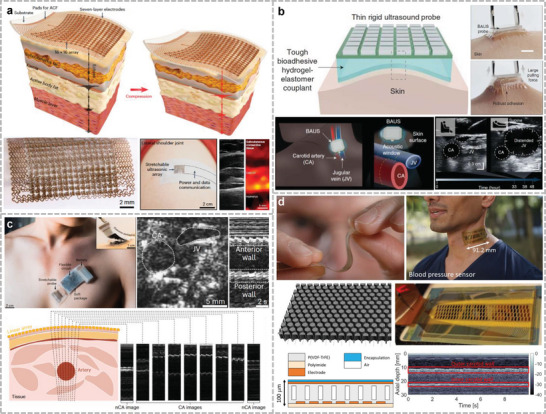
Ultrasonic imaging for deep tissue. a) Noninvasive elasticity measurements of tissues up to 4 cm subcutaneously by stretched ultrasound arrays and comparative validation with MRI. Reproduced with permission.^[^
